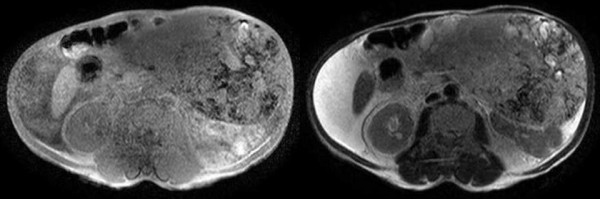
Abdominal MRI shows huge inhomogeneous mass lesion can be identified in the right side of the intra-abdomen.
